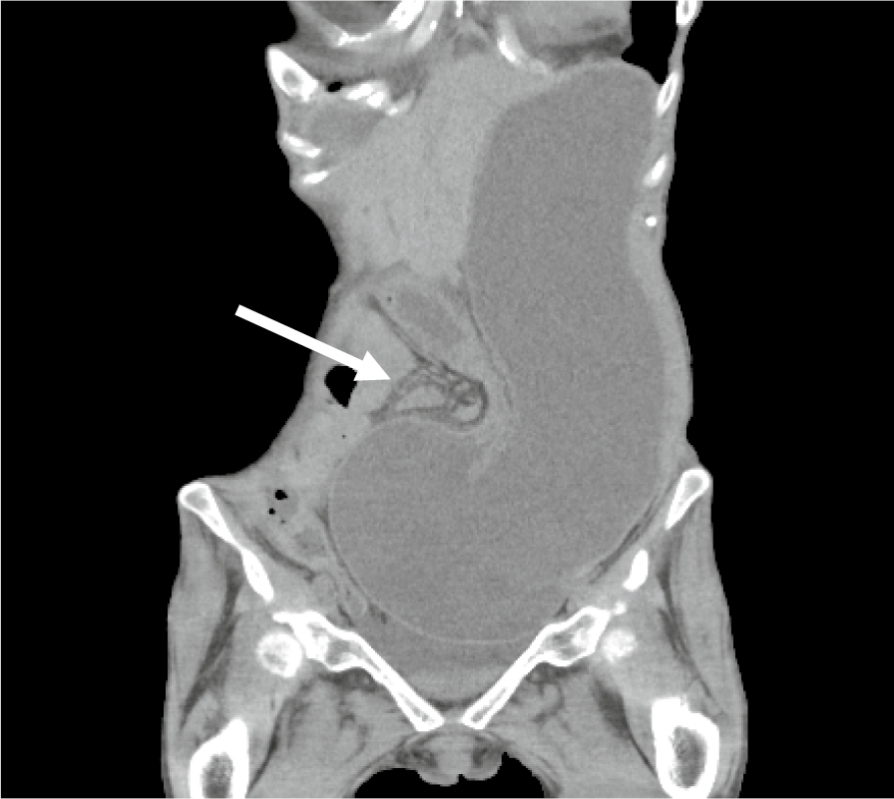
CT showed the stomach was markedly dilated due to the obstruction near the pylorus caused by the lifted sigmoid colon. A white arrow points to the sigmoid colostomy
